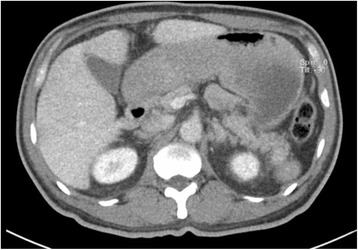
Abdominal computed tomography showing distended stomach with large amounts of gastric content in spite of 4 hours of fasting prior to the scan
